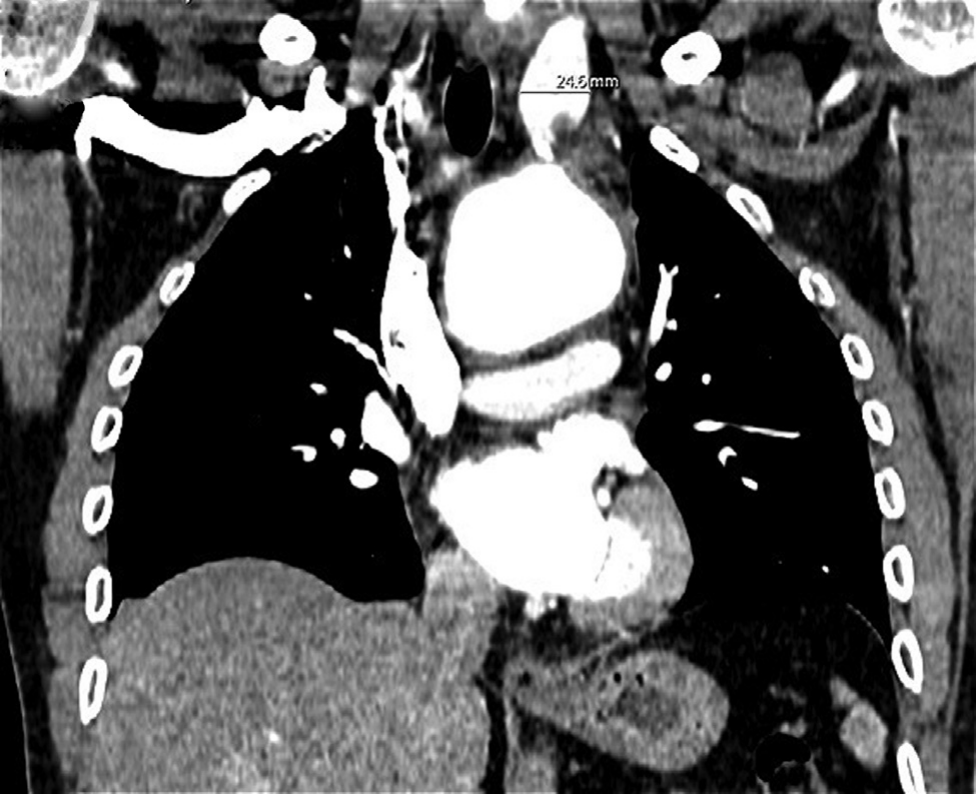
Coronal view of preoperative computed tomography scan demonstrating a 55-mm aortic arch aneurysm involving the origin of the supra-aortic trunks, with a separate aneurysm of the left common carotid (25 mm).
